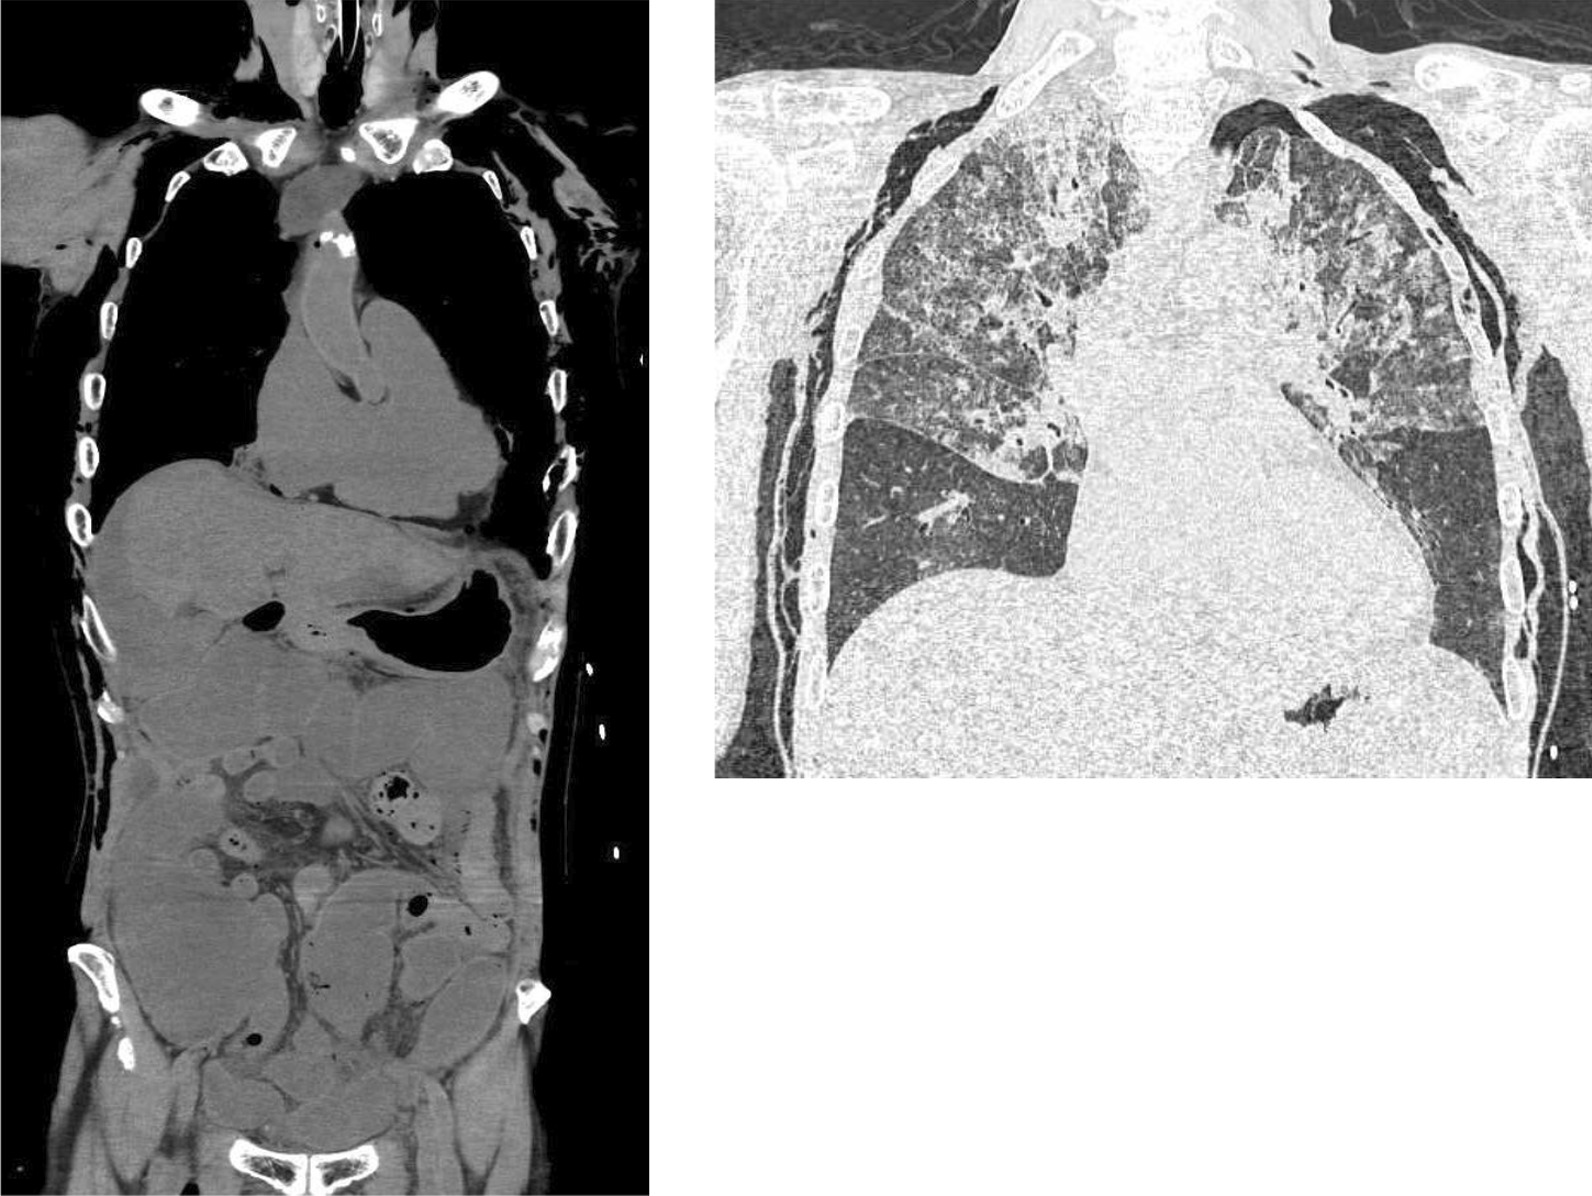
Computed tomography (CT) imaging during autopsy did not show significant changes, such as bowel perforation in the abdomen, from admission. Mild cardiomegaly, intratracheal fluid, and upper and dorsal infiltration of the lungs were observed
